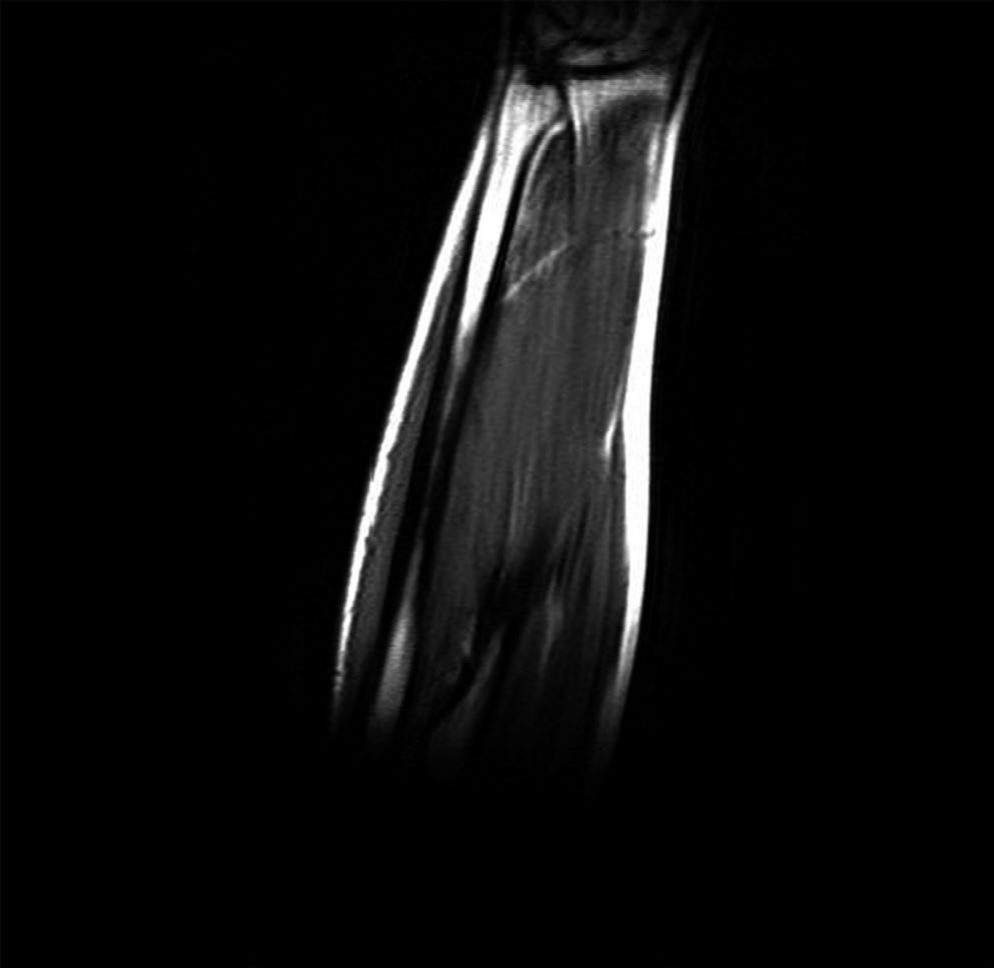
MRI without contrast, T1 image, coronal view
